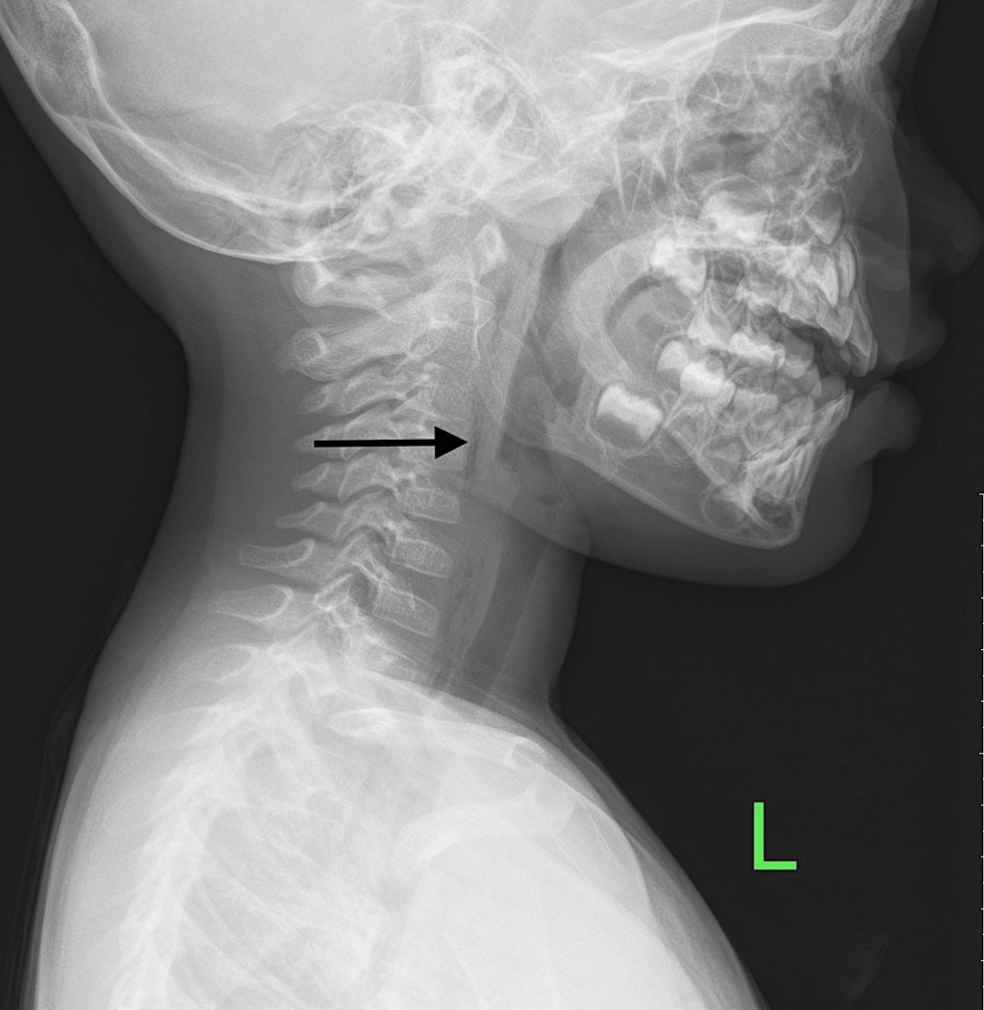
Day two: lateral soft tissue radiography of the neck showed improvement, however, there was incomplete resolution of the retropharyngeal air
